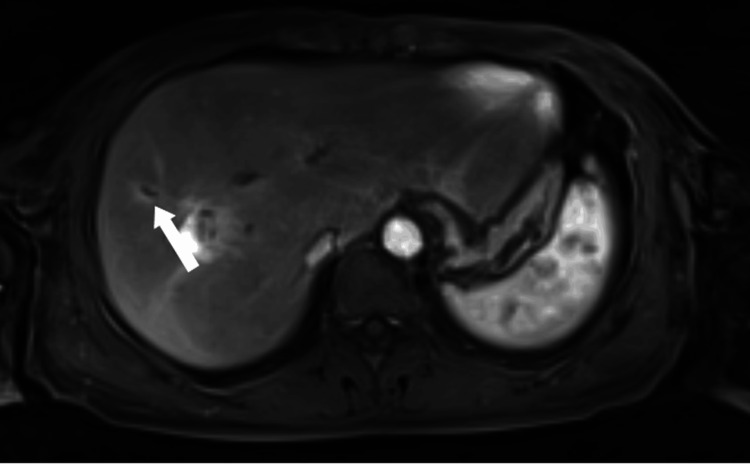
Axial contrast-enhanced MRI of the liver in the early arterial phase Contrast-enhanced MRI showing periductal enhancement in other parts of the liver, suggesting an associated inflammatory biliary process (white arrow).
